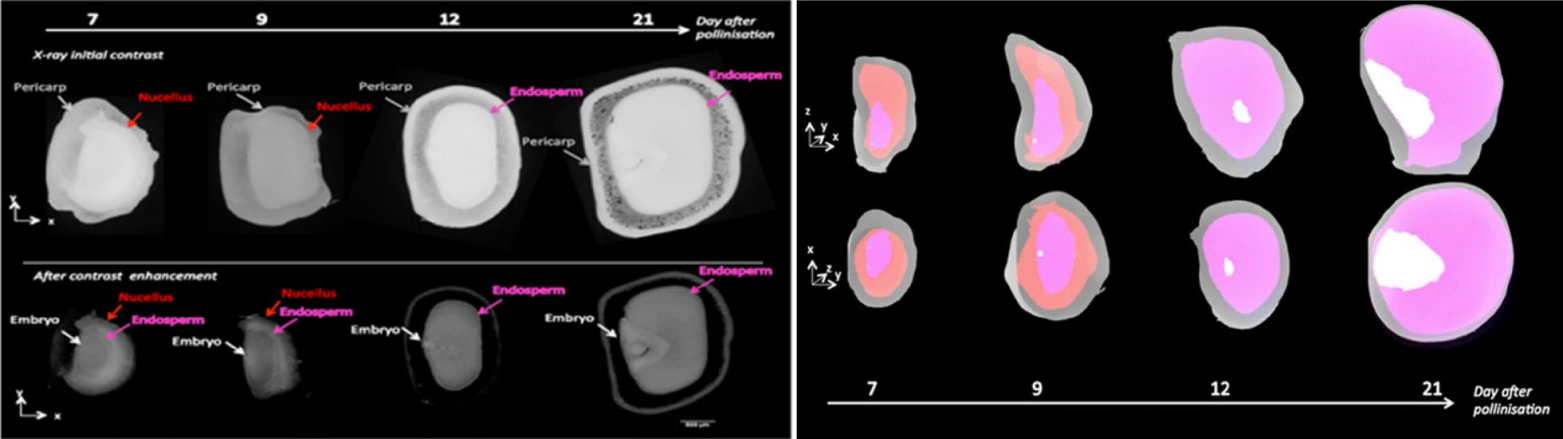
Left: X-ray images and Right: 3D segmentation of maize seeds at four different developmental stages corresponding to 7, 9, 12 and 21 days after pollination [26]
